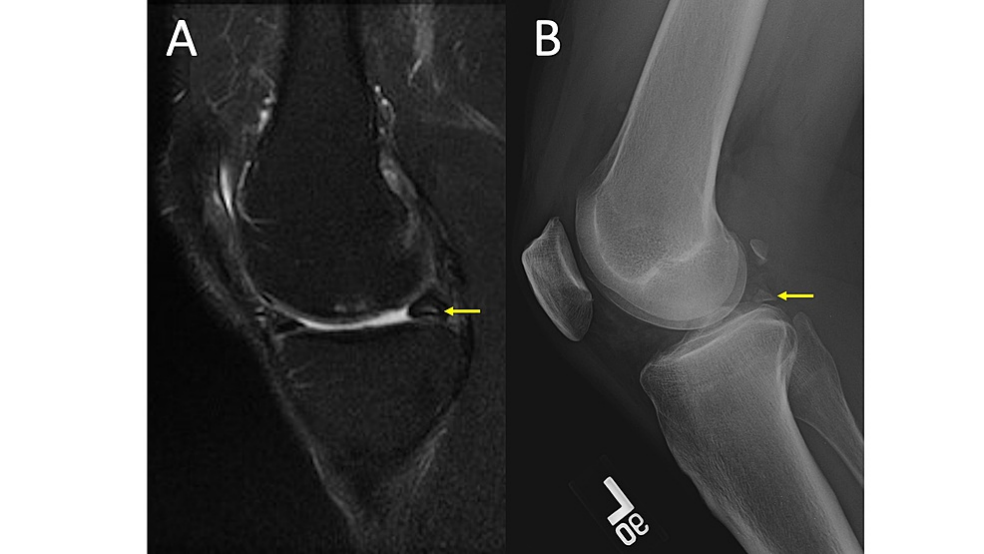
Meniscal Ossicle MRI coronal T2 fat saturation (FS) (10A) shows intermediate to hyperintense T2 signal noted within the posterior horn of the medial meniscus (yellow arrow), which may be interpreted as a meniscal tear or intrasubstance degeneration. A lateral knee radiograph (10B) demonstrates an ossified triangular-shaped body within the posterior joint space (yellow arrow) revealing the apparent meniscal tear or intrasubstance degeneration to be a meniscal ossicle.
